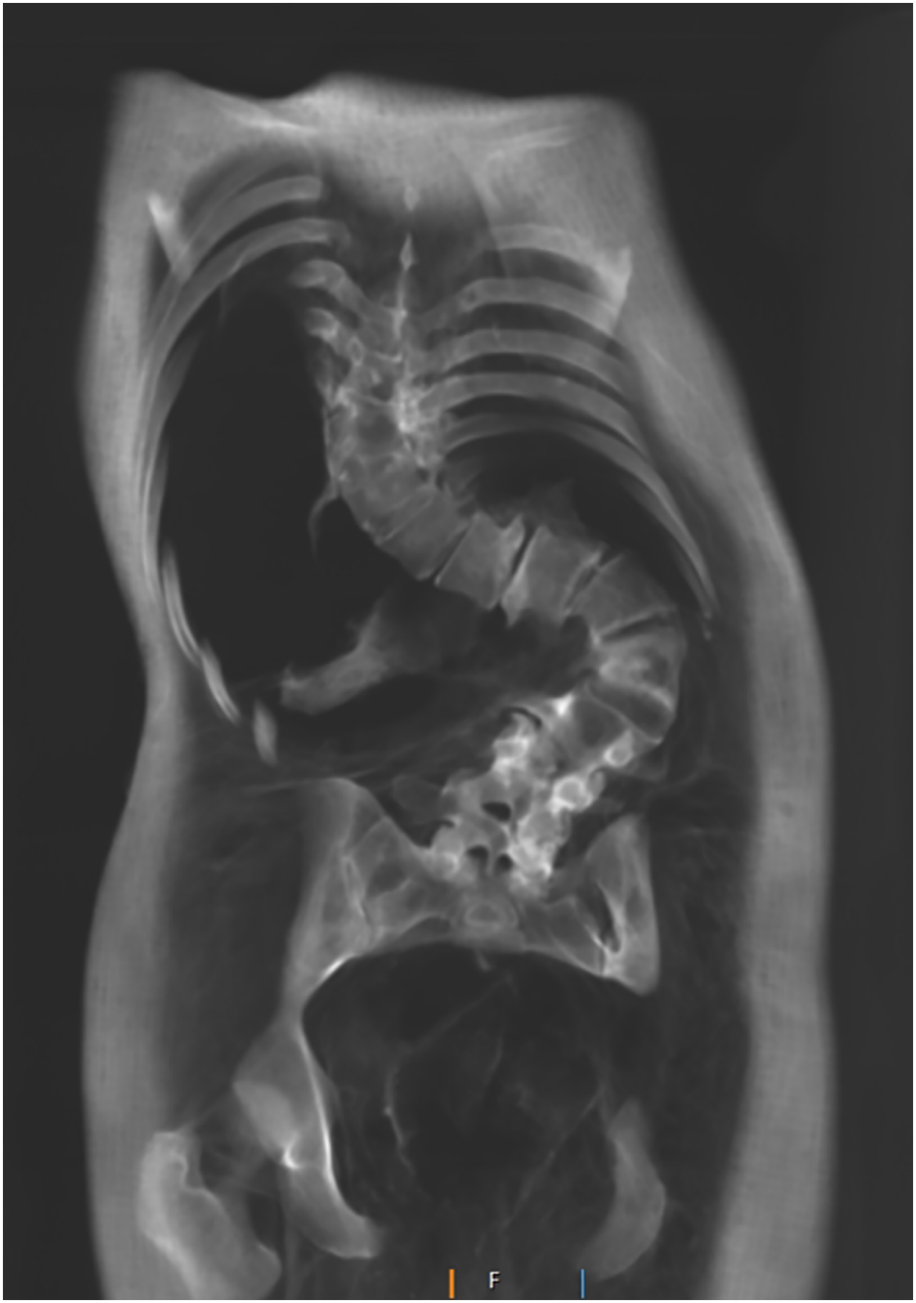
Coronal multi-planar reconstruction of a CT data set depicting the spine of individual A with severe scoliosis and secondary degenerative changes.
